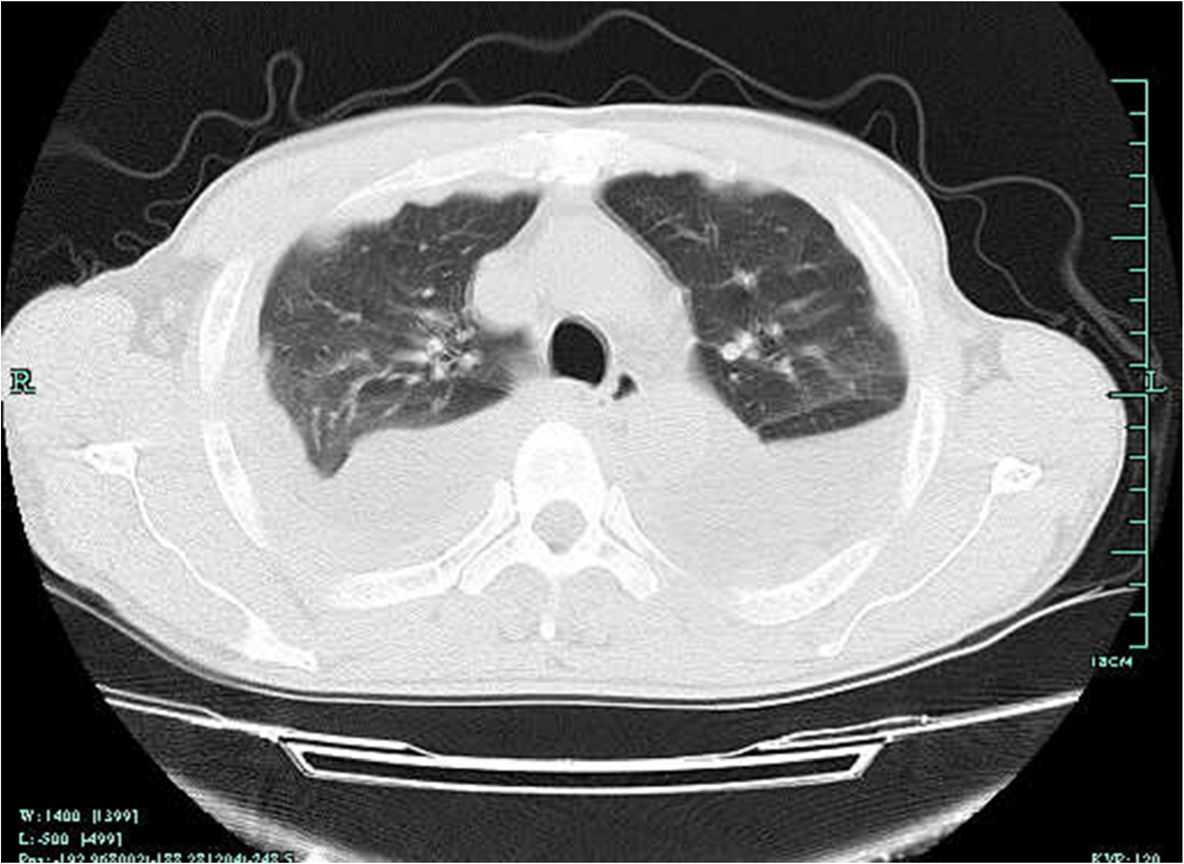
CT scan of the thorax. CT scan of the thorax showing a bilateral pleural effusion, diffused pleural thickening and multiple nodular lesions in the parietal pleura.
